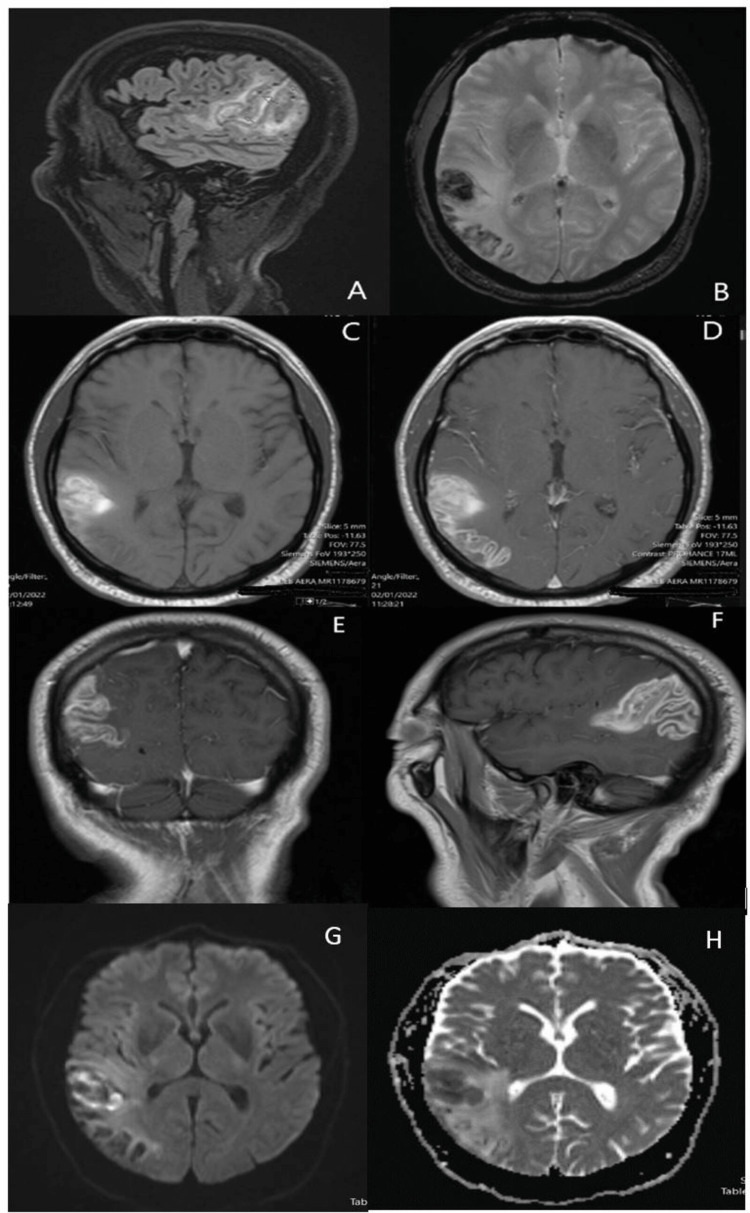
MRI findings Fine, planar hyperintensity (arrows) seen on 3D Flair from dorsal cephalad to ventral supermarginal gyrus (A), T2-weighted hemosiderin view (B) of hemorrhage, axial T1-view of insult on dorsal one-third of temporal gyrus, dorsal posterior ramus of supramarginal gyrus, and angular gyrus pre- (C) and post- (D) contrast, showing hypointensity in areas with vasogenic edema. T1 post-contrast coronal view (E) and sagittal view (F) showing vasogenic edema surrounding hemorrhage in superolateral occipital lobe, with hemorrhage extending along gyrus without intracerebral extension. DWI images showing hyperintensity with surrounding edema (G) and ADC (H) showing R-sided edema extending intracortically.
